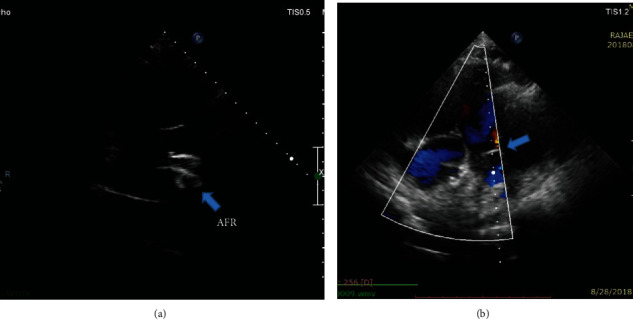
Follow-up echocardiography indicating the proper position of the AFR device with left-to-right shunt.
